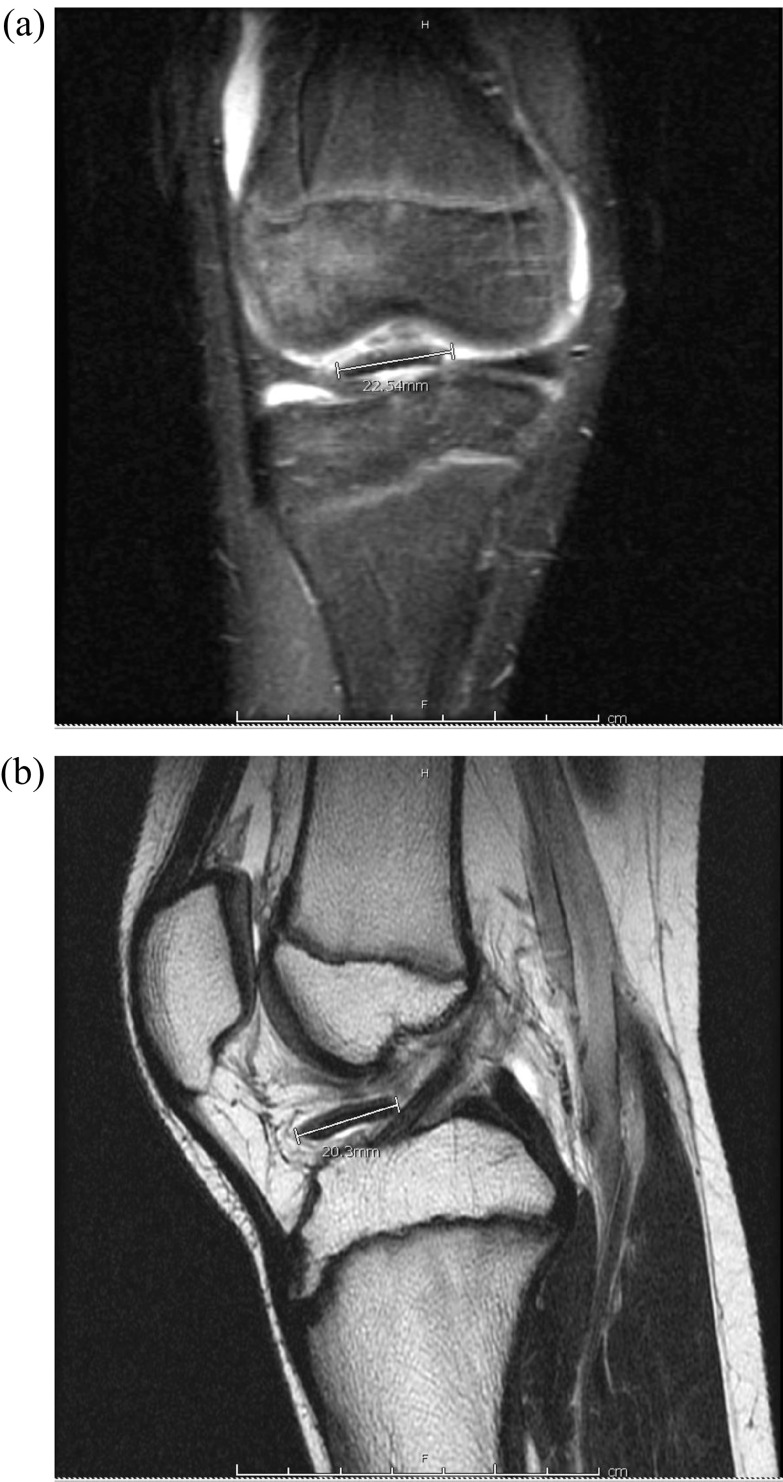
T2- and T1-weighted MRI demonstrating large chondral fragment within the intercondylar notch (a and b).
